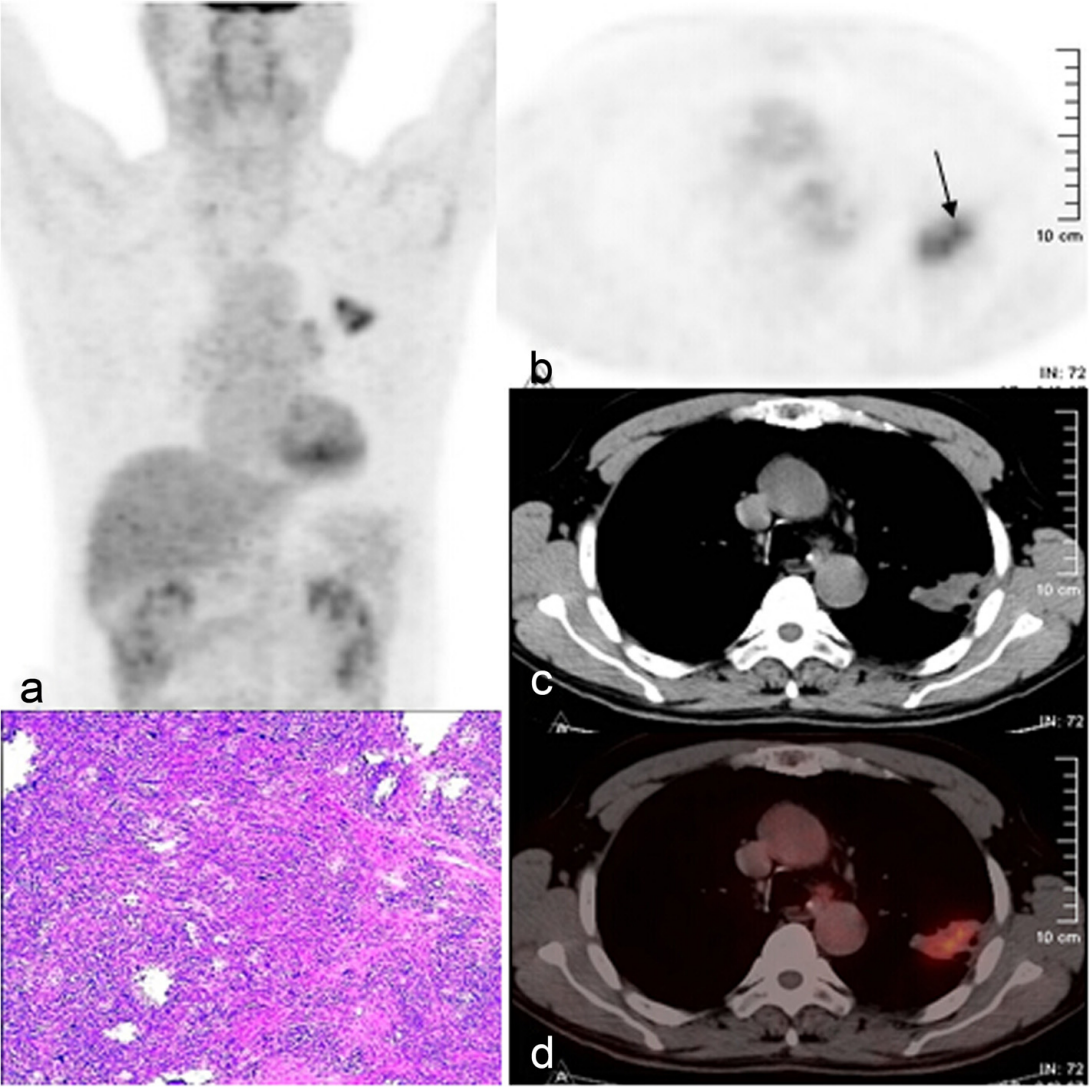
Multi-focus ^18^F-FDG uptake mass in a 65-year-old male. The whole-body PET (a) and axial PET (b), CT (c), and fused PET/CT images (d) show multi-focus FDG accumulation in the mass of the left upper lung lobe (*black arrow*). The pathologic result was inflammatory pseudotumor
